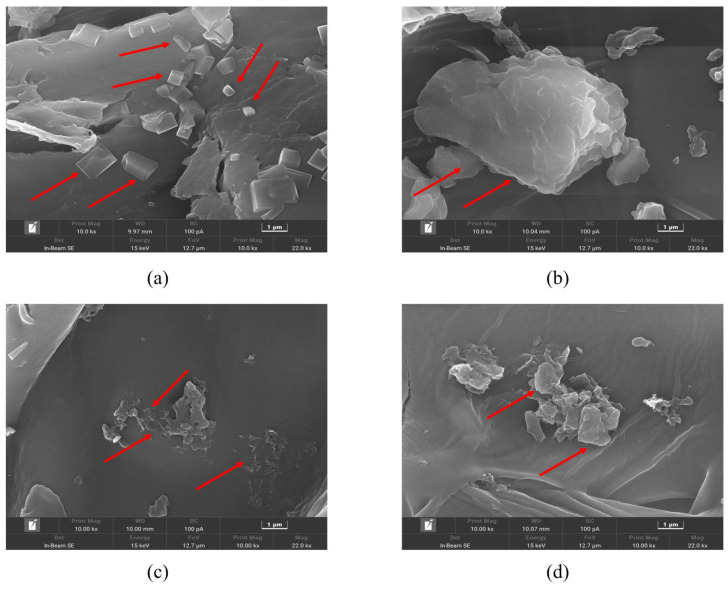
SEM images of leaf cell residues of hot air-dried Pandan powder and freeze-dried Pandan powder before and after ultrasound-assisted extraction at 10,000 magnifications disrupted. Differences can be seen where the red arrows point. (a) hot air-dried and (b) freeze-dried Pandan powder before extraction; (c) hot air-dried and (d) freeze-dried Pandan powder after extraction.
